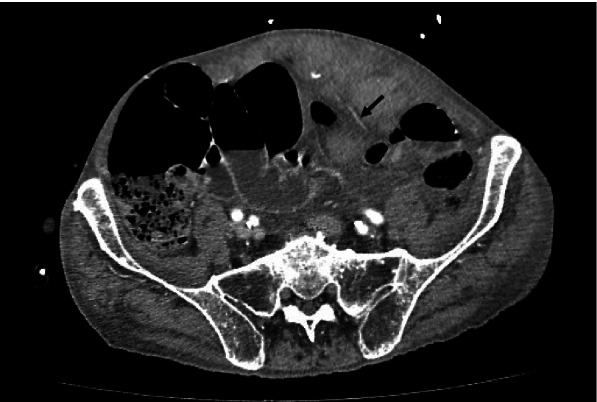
Contrast CT abdomen: Prominent serpiginous enhancing structures within (arrow) representing prominent tortuous vessels. Contrast extravasation was absent
